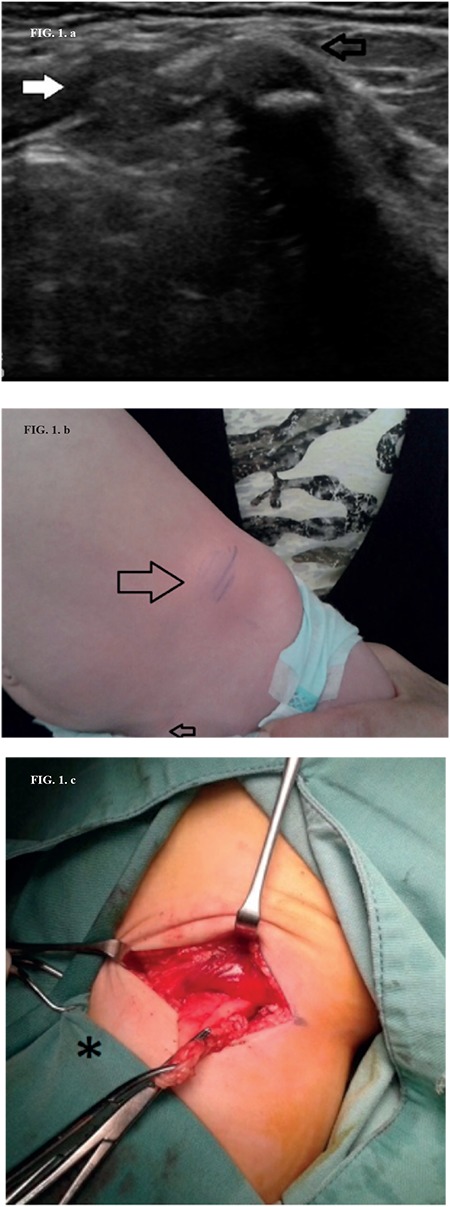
Ultrasound assay: Preperitoneal fat and bowel herniating through the transversalis fascia (white arrow). Iliac crest (black arrow) (a). Preoperative presentation of the Spigelian hernia (large arrow). Left inguinal hernia operative scar (small arrow) (b). Preperitoneal fat and peritoneum herniating through the transversalis fascia. Left inguinal incision site (asterisk) (c).
